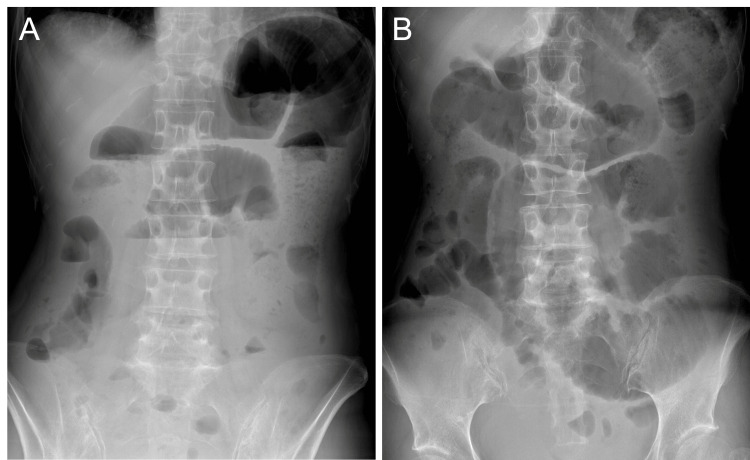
Abdominal radiography showing small bowel dilation with multiple air-fluid levels (A) Abdominal radiograph in the standing position demonstrating air-fluid levels. (B) Abdominal radiograph in the supine position, highlighting bowel dilatation
